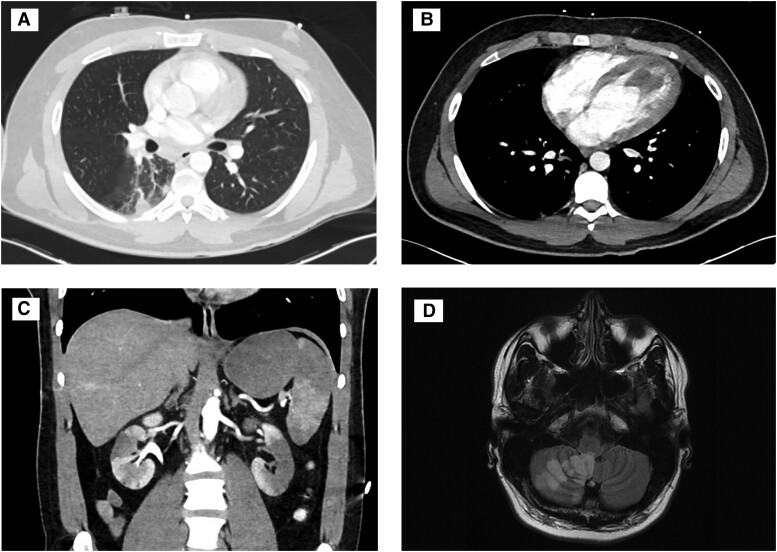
Diagnostic imaging findings. Computed tomography angiography of the chest revealing (*A*) patchy pulmonary opacities, (*B*) apical left ventricular filling defect with hypoattenuation of the apical wall, (*C*) computerized tomographic abdomen and pelvis with areas of hypoattenuation in the kidneys and spleen consistent with embolic infarction, and (*D*) brain magnetic resonance imaging revealing acute infarct of the right inferior cerebellar hemisphere.
